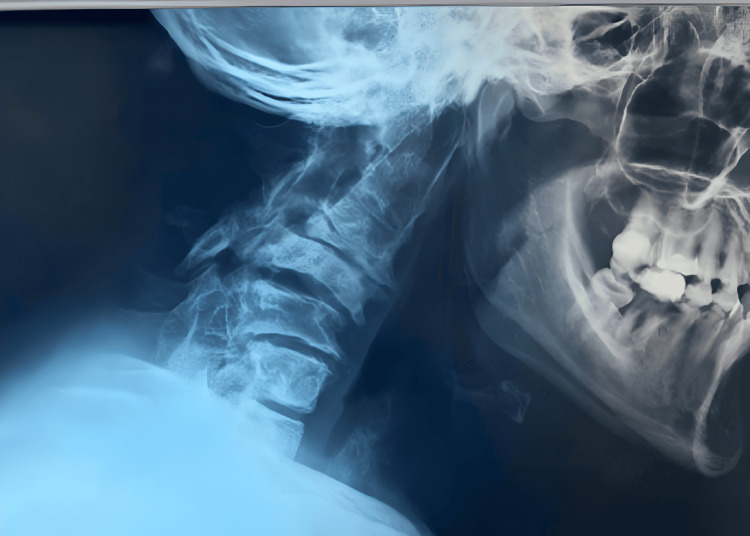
Preoperative lateral view of radiograph of the cervical spine.
